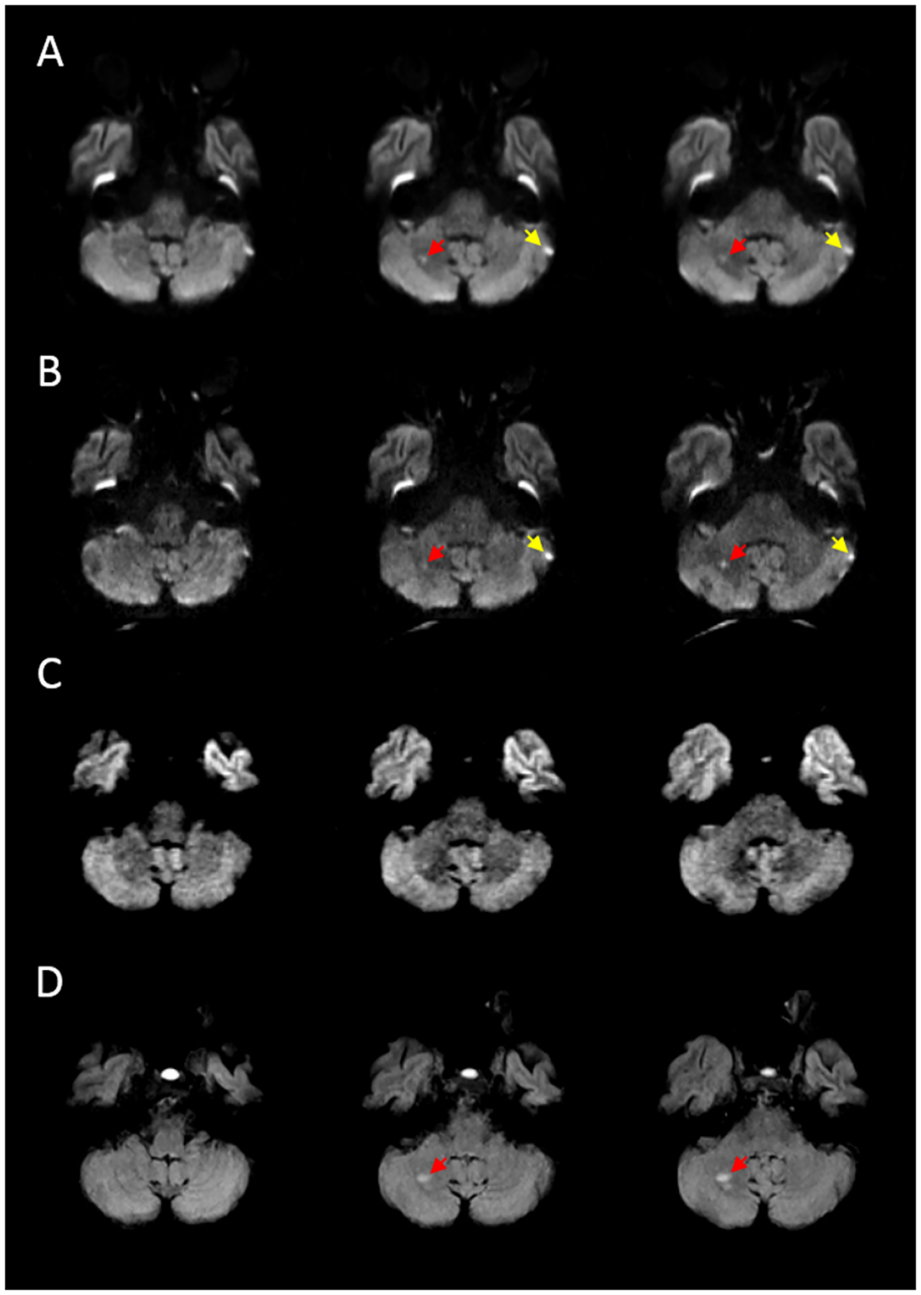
Example of disagreement between the DWI sequences. In this patient, MRI was performed 25 hours after the onset of stroke symptoms. Both low (A) and high-resolution (B) EPI-DWI show a hyperintensity (red arrows) in the right cerebellar lobe that was judged to be an ischemic lesion. Also note the hyperintensity in the periphery of the left cerebellar lobe (yellow arrows), which was judged to be an artifact. C) STEAM-DWI was judged as normal in this case. D) FLAIR performed 5 days after baseline scans confirmed the presence of an infarct in the same location.
